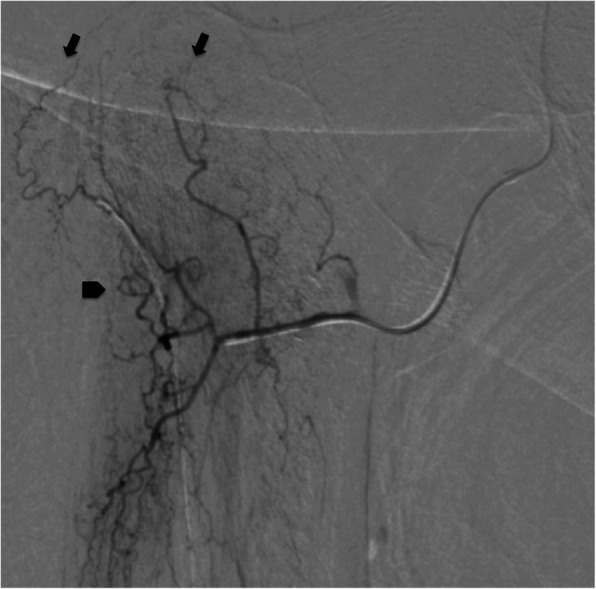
Super selective angiography of the ascending branch of the lateral femoral circumflex artery with a 2.4Fr micro catheter. The “inflammatory blush” was not found, however, small corkscrew arteries ascending to he point of hipersignal in the CT were found (arrows). There were a few more corkscrew-shaped arteries in a point of pain during the procedure (arrowhead), which were also occluded
